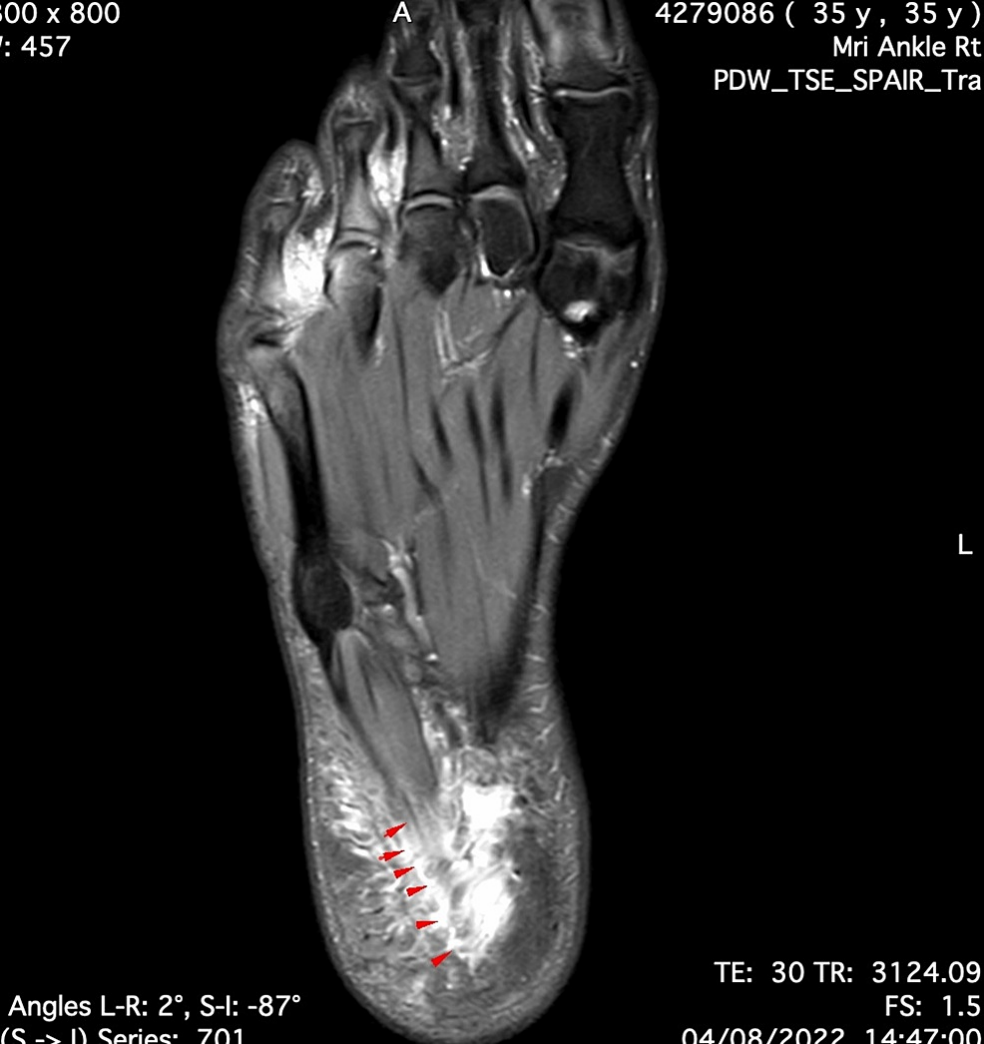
Axial cut of proton-density fat-saturated MRI. Tear of the proximal segment of the lateral band of the plantar fascia, which appears with a bright signal (red arrows). Note the edema in the surrounding tissues.
